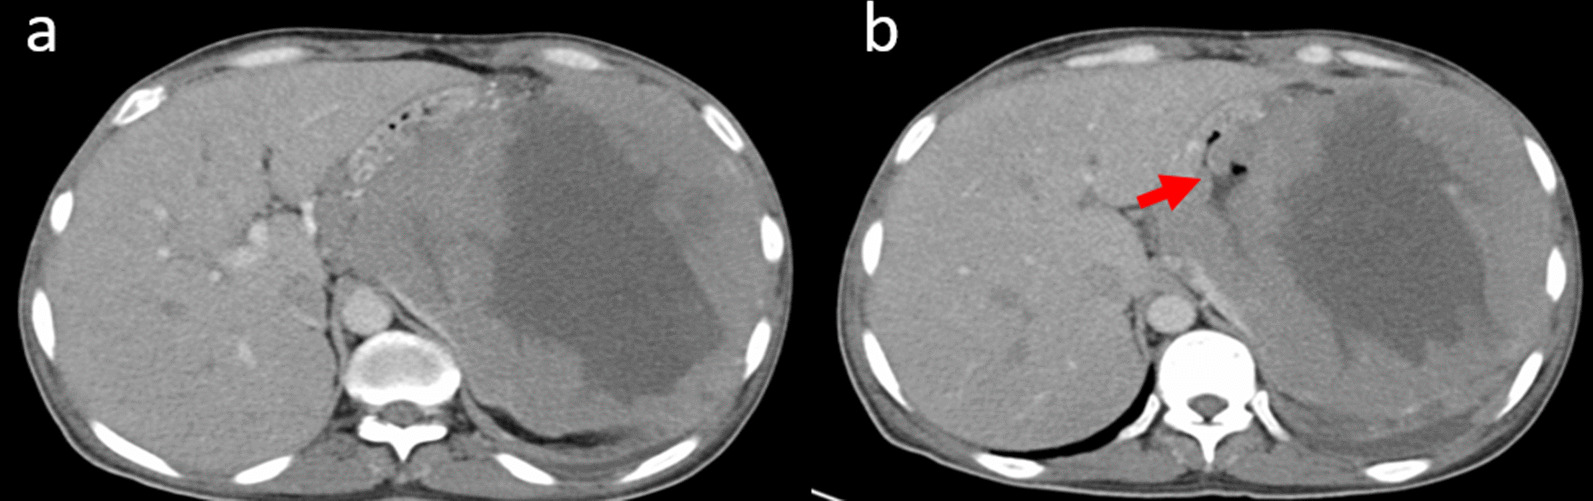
Computed tomography scan views demonstrating a large necrotic splenic mass (a) communicating with the stomach via a gastrosplenic fistula (b, red arrow)
